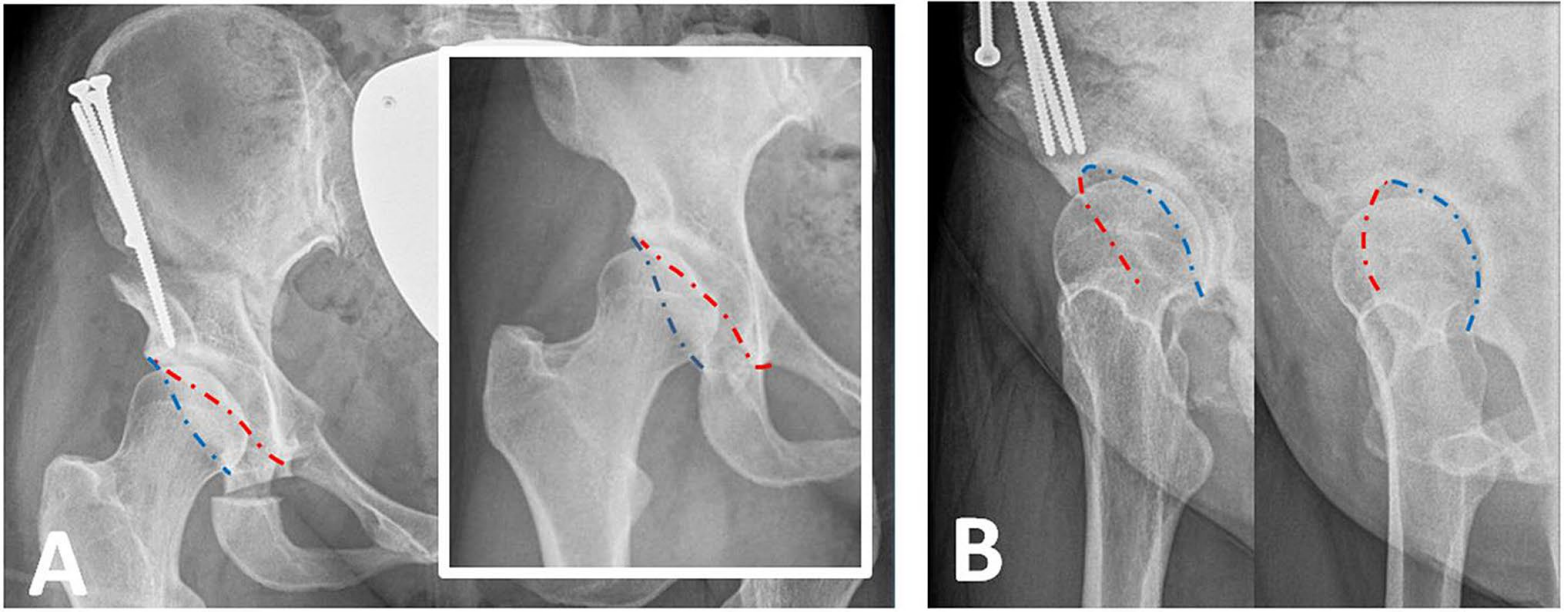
Pre and postoperative X-ray of a female dysplasia following periacetabular osteotomy in anteroposterior view (a) and faux profile view (b). The dashed line shows the anterior (red) and posterior (blue) acetabular rim
